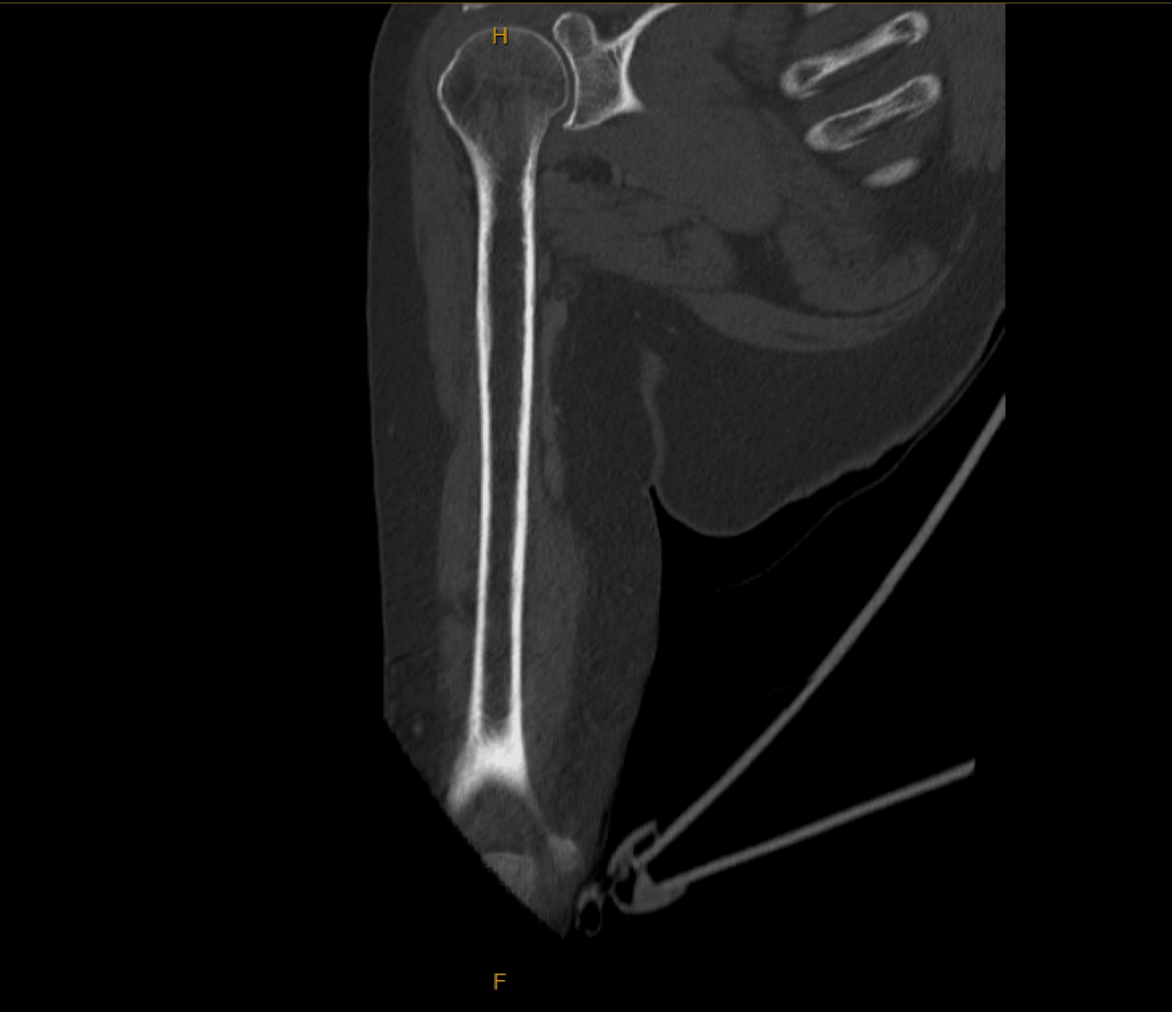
Coronal CT Bone Window Image of the Right Humerus With IV Contrast Coronal CT image of the right humerus in bone window (with IV contrast), demonstrating preserved alignment of the glenohumeral joint with mild-to-moderate joint space narrowing and subchondral changes consistent with osteoarthritis. The humeral head and glenoid are intact with no evidence of fracture, dislocation, or osseous lesion. The acromioclavicular joint is partially visualized superiorly and appears moderately degenerated. The visualized bony structures of the humeral shaft are intact, with no cortical disruption.
